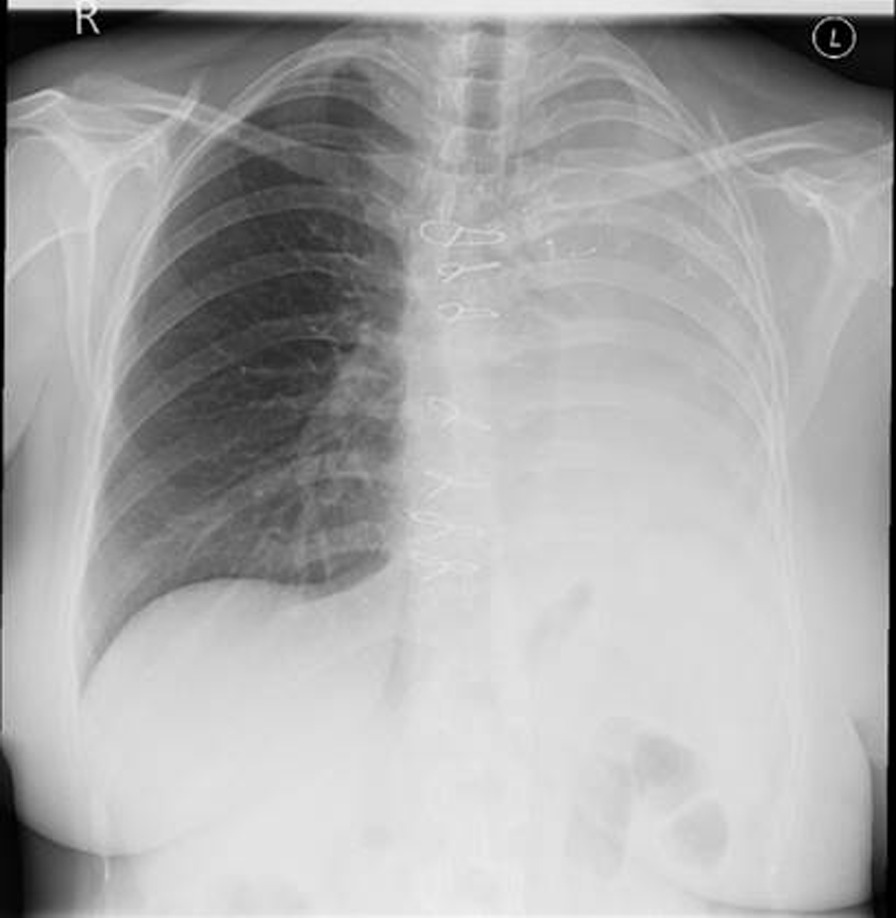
Chest x-ray one month post hospital discharge from August 2022 (re-collapse of upper lobe)
